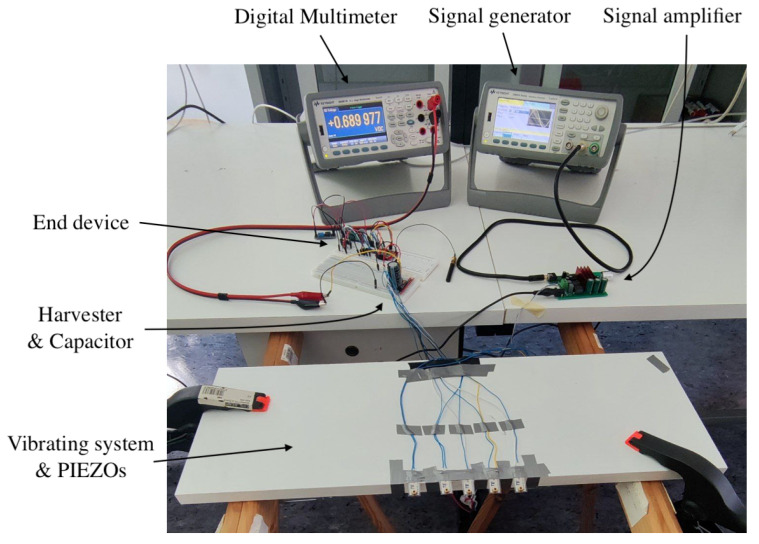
Complete setup: vibrating subsystem, signal generation and amplification subsystem, harvester and storage capacitor, end device with ultrasonic sensor embedded.
